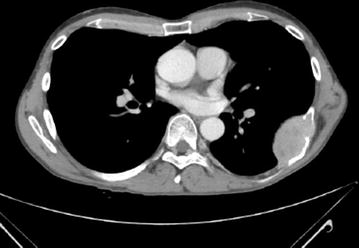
Single bone metastasis from meningeal SFT (patient 1 in Table 1): CT scan (venous phase after contrast medium) shows a solid lesion characterized by homogeneous contrast enhancement at the level of the seventh left rib
